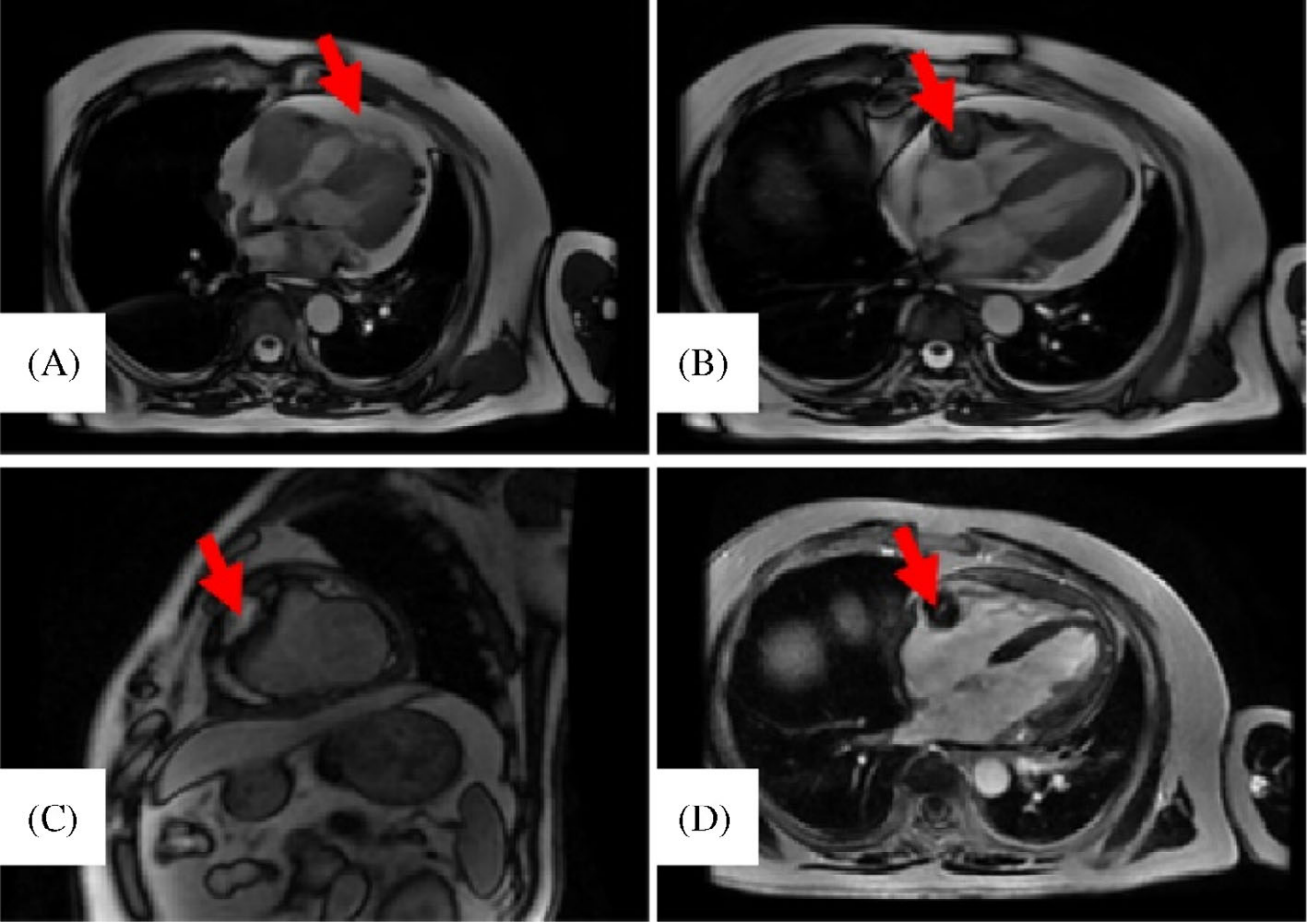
Imaging revealed cardiac metastases. (a) A small amount of pericardial effusion was detected. (b) Cardiac magnetic resonance imaging showed a mass (36 mm × 24 mm × 54 mm) with higher signal intensity on T2 sequences, invading the right coronary arteries and pericardium. (c) The mass showed markedly enhanced signal in myocardial resting perfusion imaging. (d) The mass had hypointense in delayed enhanced imaging.
